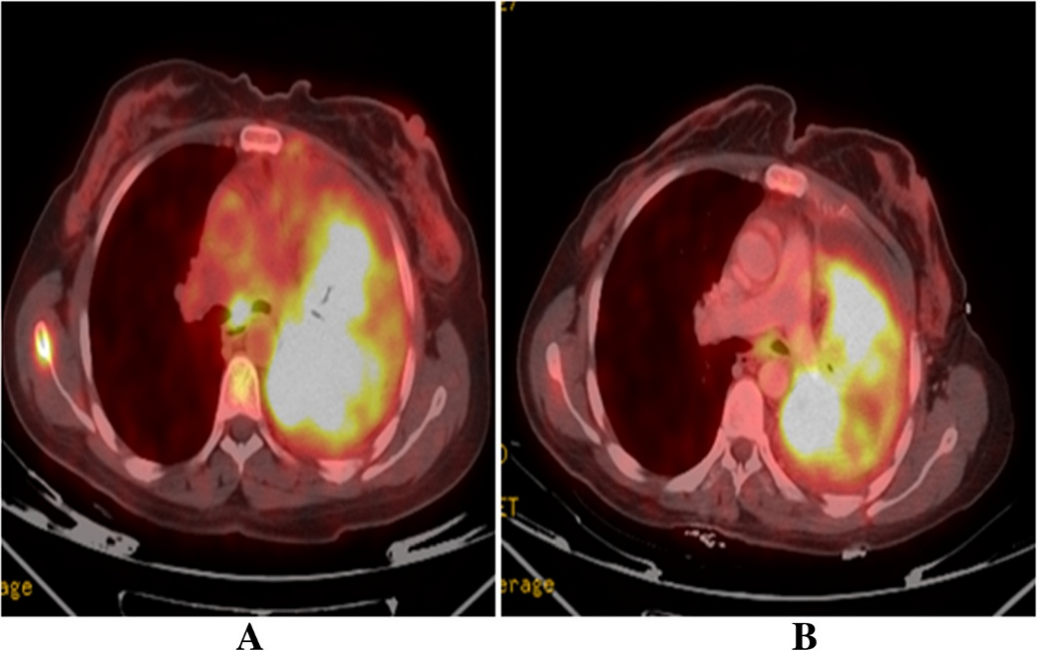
PET-CT scan before (A) and after (B) three months of treatment with erlotinib, showing improvement in the mediastinal lymph node and left lung uptake.
